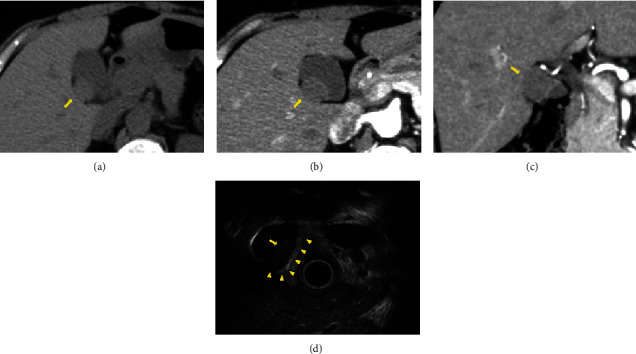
(a) Plain phase of CT showed a low-intensity lesion in the neck of the gallbladder (arrow). (b, c) Arterial phase of the CT revealed a lesion that was contrasted in the neck of the gallbladder, which has an unclear border with the liver (arrow). (d) Endoscopic US showed an isoechoic lesion on the intraperitoneal side of the gallbladder (arrow), without any breakage in the gallbladder wall (arrowhead). CT: computed tomography; US: ultrasonography.
